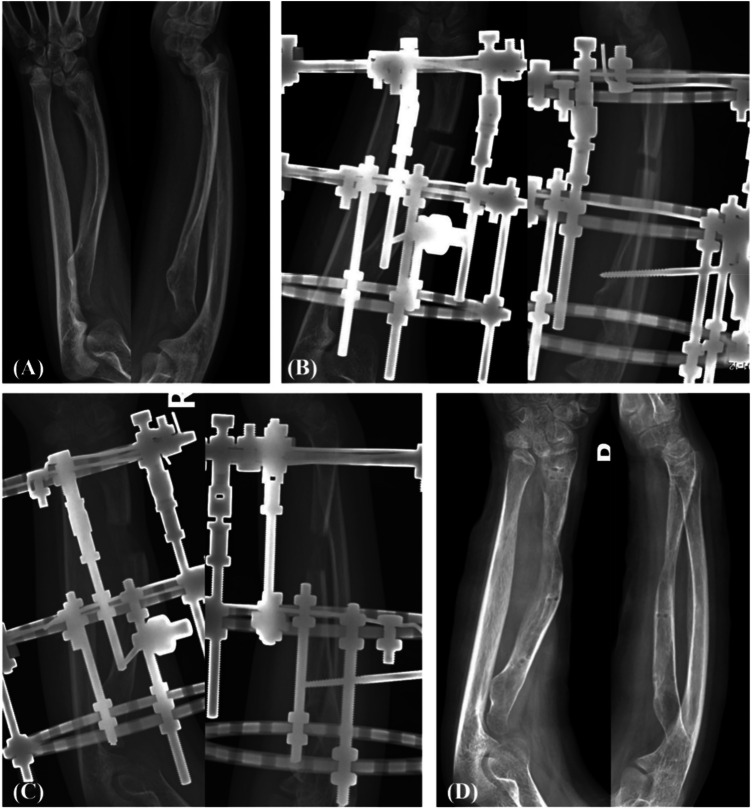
A 13-year-old female patient with right Madelung deformity. (A) Preoperative anteroposterior and lateral x-rays. (B) Thirteen days after Ilizarov application, lengthening was in progress. (C) Two months postoperation, lengthening was complete and ossification at the osteotomy site was visible. (D) Five months postoperation, full ossification was achieved and the external fixator was removed. At 4-year clinical follow-up, the patient maintained full, painless wrist function with no recurrence of the deformity.
